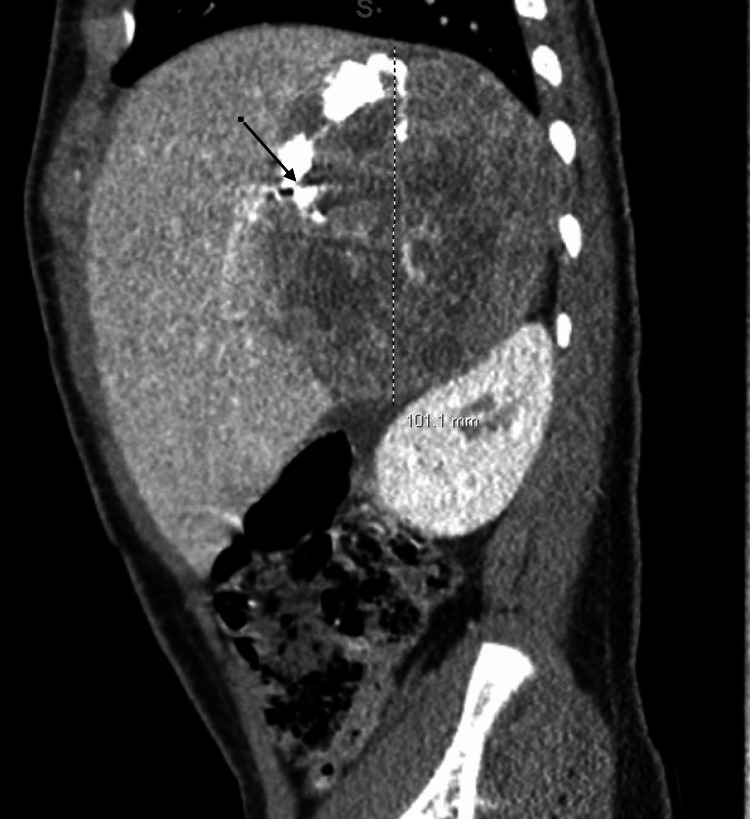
CT abdomen/pelvis with PO/IV contrast: large heterogeneously enhancing right suprarenal mass measuring approximately 10.1 x 8.7 x 8.4 cm.
